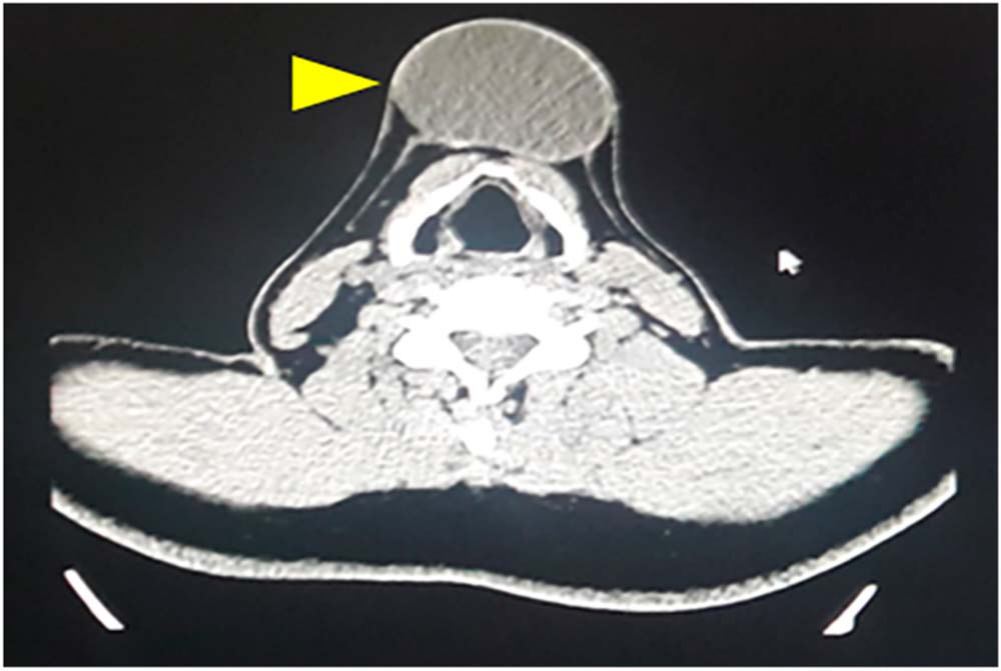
Contrast-enhanced CT scan of the neck showing an encapsulated cystic lesion.
